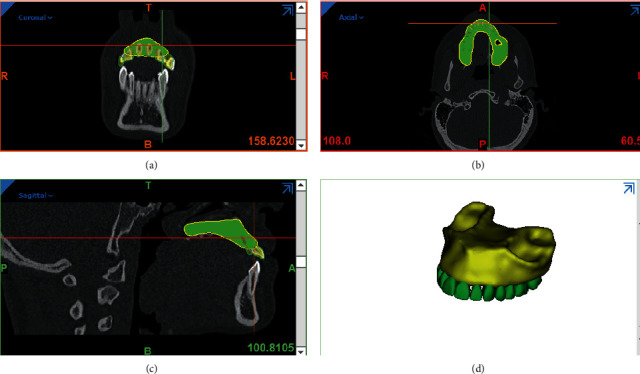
Segmentation of bones and teeth in the CT scan volume.
